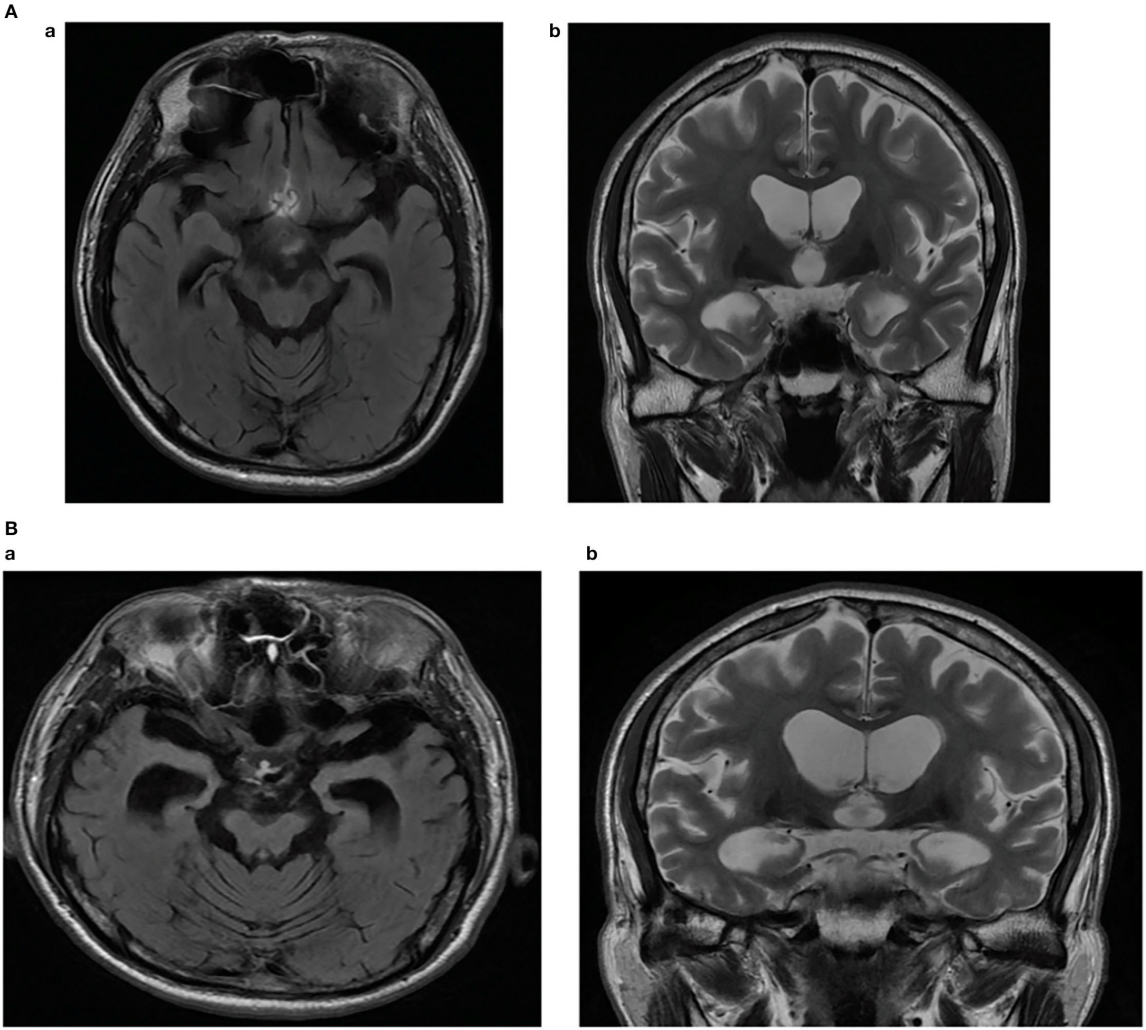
(A) Brain magnetic resonance imaging of the patient showing diffuse atrophy of the brain, more predominant medial temporal lobes. Fluid-attenuated inversion recovery (FLAIR) axial image (a), T2-weighted coronal image (b). (B) Brain magnetic resonance imaging (MRI) of the patient showing interval progression of diffuse (mainly frontotemporal) brain atrophy and marked medial temporal lobar atrophy after 18 months. Marked increased in size of with signal void in cerebral aqueduct, decreased in size of both basal ganglia compared to the MRI of the first visit are revealed. Fluid-attenuated inversion recovery (FLAIR) axial image (a), T2-weighted coronal image (b).
